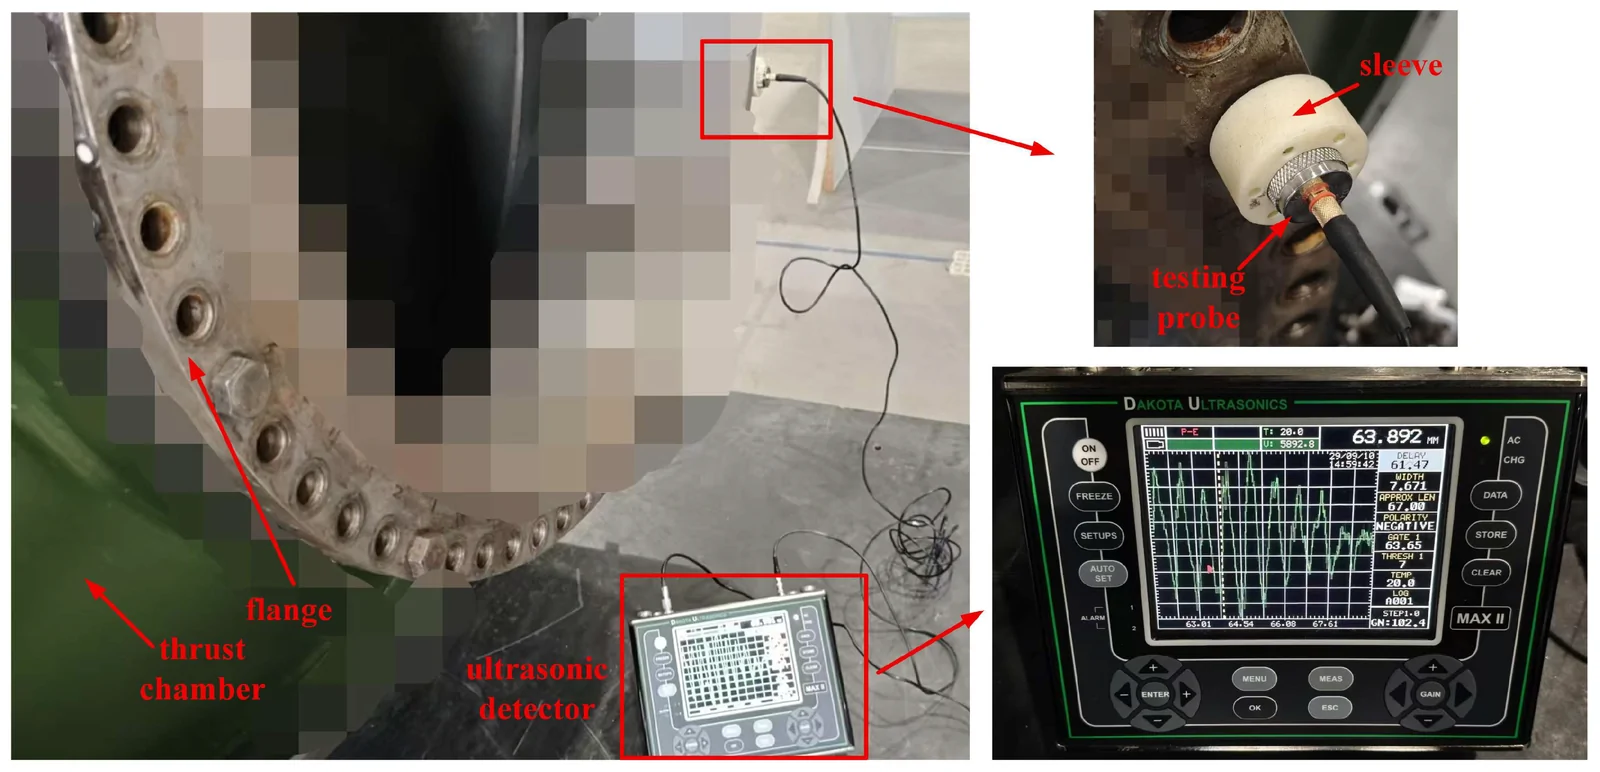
Ultrasonic preload measurement setup with a positioning sleeve, bolt-axis propagation path, and measurement direction.
